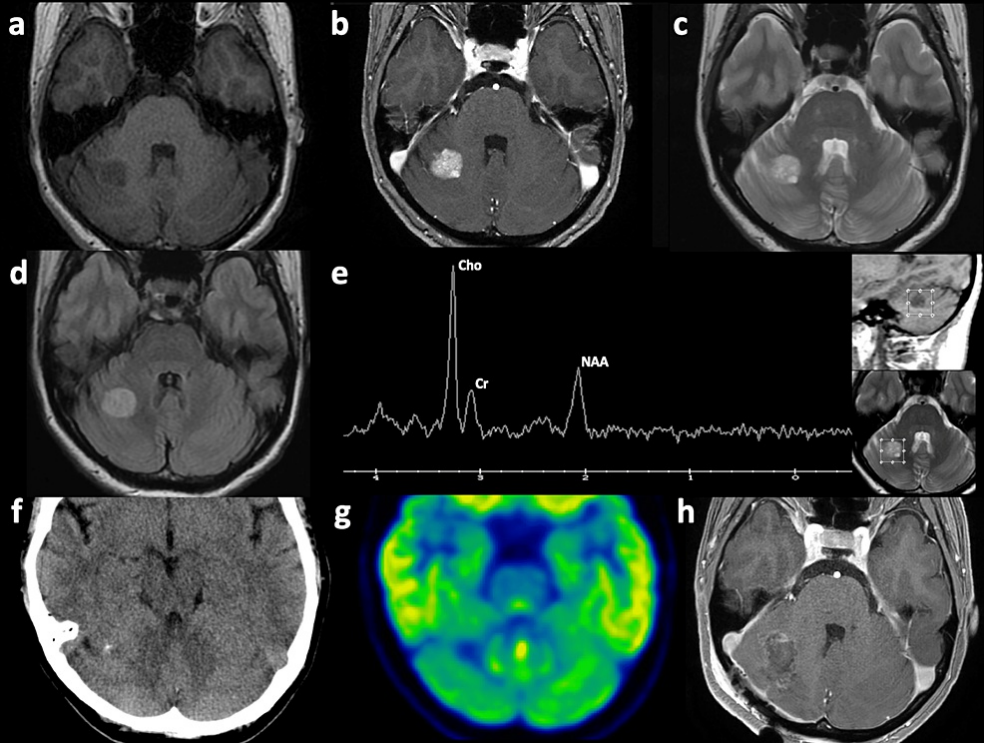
Pre- and postoperative images. T1WI of the brain shows a low-intensity intra-axial tumoral lesion in the right cerebellum (a), with heterogeneous gadolinium enhancement (b). T2WI (c) and FLAIR (d) show a heterogeneously hyperintense tumor without PTBE. (e) MRS shows peaks of Cho, Cr and NAA with a high ratio of Cho/NAA. (f) CT shows partial calcification in the tumor. (g) FDG-PET shows hypo-accumulation at the tumoral lesion with an L/N ratio of 0.50. (h) Postoperative gadolinium-enhanced T1WI of the brain shows gross total resection of the tumor.
